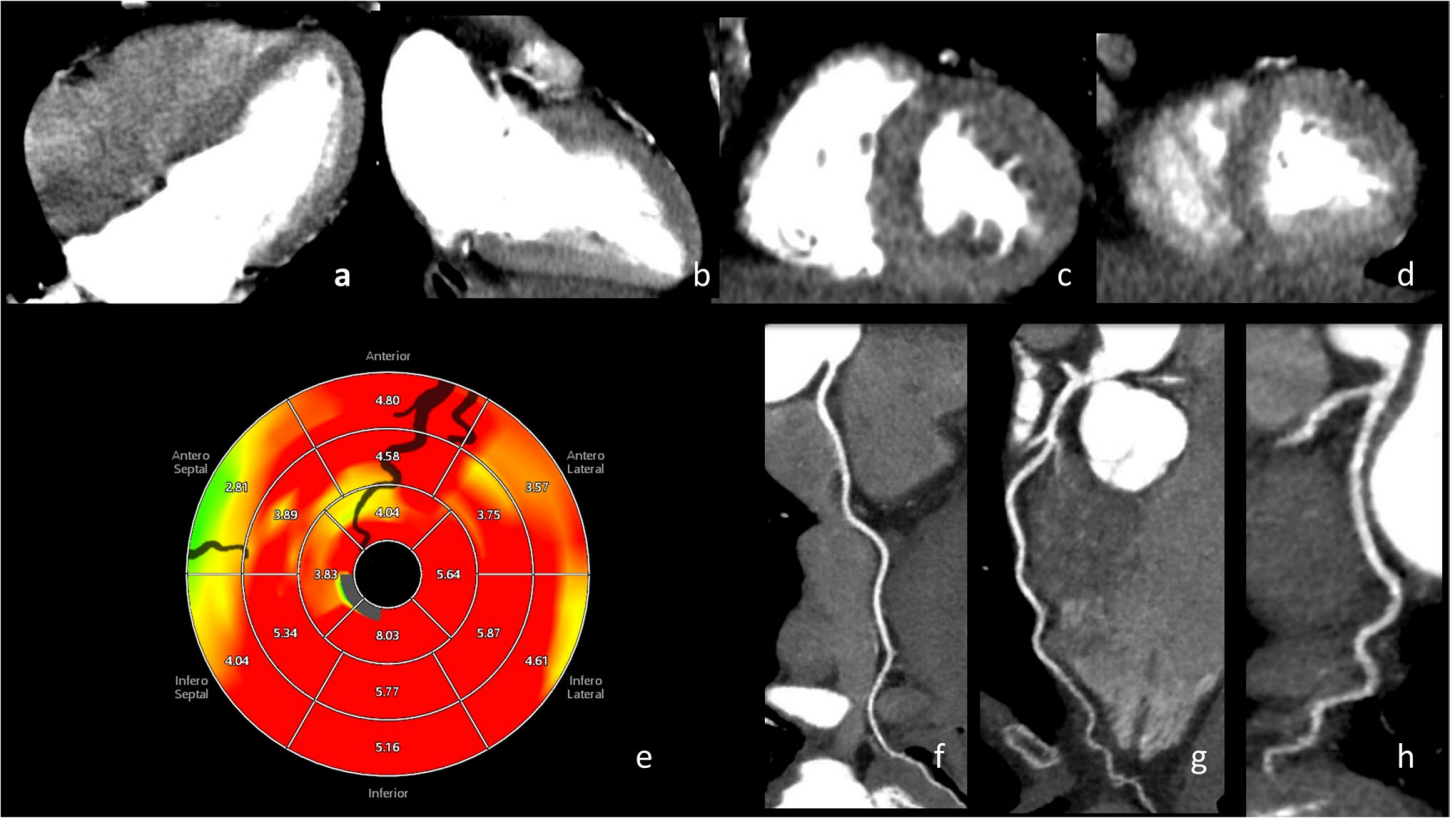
A 59-year-old female ex-smoker with familial hypercholesterolemia and relevant family history of cardiovascular disease presented to the hospital with atypical angina pectoris for the first time. She was referred for stress dynamic myocardial CT perfusion with FFR-CT. The coronary CTA showed no calcifications in the coronary arteries (RCA – f, LAD – g, LCX – h) or valves, except small calcifications in the aortic root and ascending aorta. In dynamic CTP: a normal stress perfusion CT images. Stress perfusion with adenosine (140 μg/kg) showed no evidence of obstructive epicardial coronary artery disease (a, b, c, d, e): no anomalies at the origin of the coronary arteries. No stenosis or plaques in the RCA, LAD, or LCX. CT-FFR with no significant pressure difference across arteries. In the CT extracardiac findings, the patient had a small thoracic hernia most probably responsible for the atypical chest pain. Upper row: dynamic myocardial CTP imaging: a four-chamber view, b two-chamber view; short axis: c basal view, d midventricular view. Lower row: e dynamic CT perfusion map with artery visual representation (black shadows), f coronary CTA RCA, g coronary CTA LAD, h coronary CTA LCX
